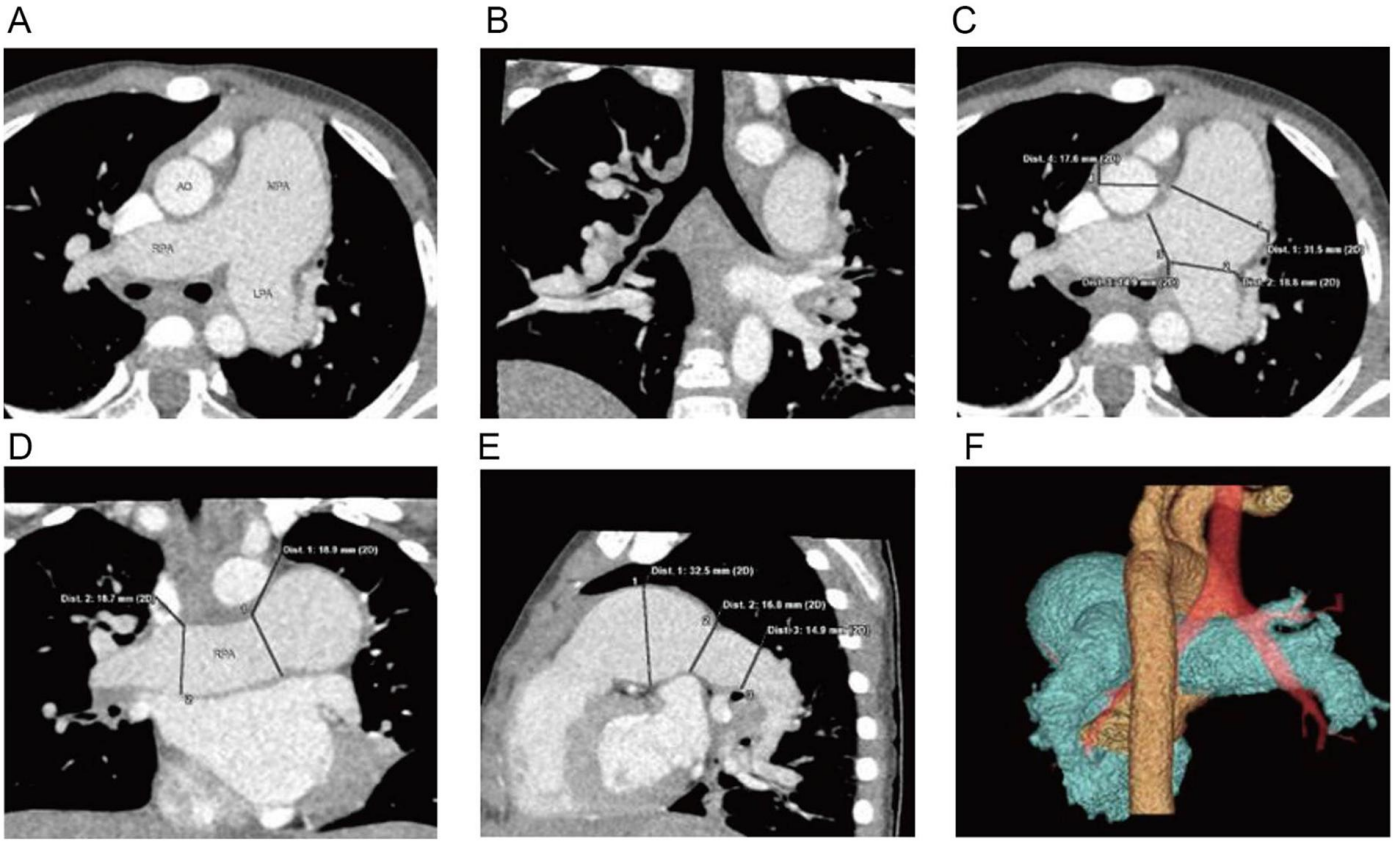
Cardiac CTA: (A) significant dilation of the main pulmonary artery and its left and right branches, (B) coronal reconstruction reveals mild narrowing of the lumen of the right lower lobe bronchus, (C–E) three-dimensional reconstructions displaying the dilated main pulmonary artery and its left and right branches, (F) volume-rendered (VR) imaging providing a visualization of the relationship between the dilated pulmonary artery, aorta, and trachea.
